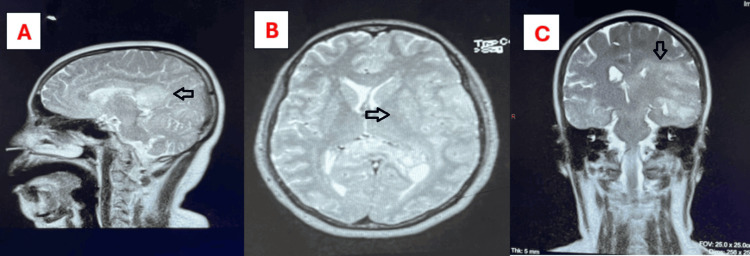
(A) Sagittal view, (B) axial view, and (C) coronal view of the T2 images of the last MRI of the brain and arrows showing multifocal lesions involving the splenium of the corpus callosum and the left cerebral hemisphere as described with associated white matter oedema and mass effect demonstrating diffusion restriction and heterogenous peripheral contrast enhancement MRI: magnetic resonance imaging
